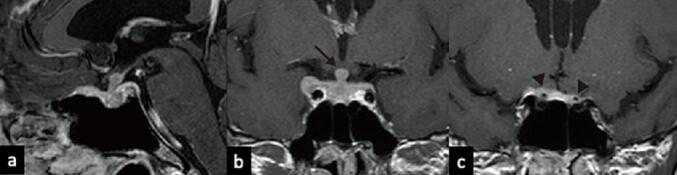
T1-weighted image with contrast before treatment demonstrates the intrasellar tumor without the optic chiasm compression (arrow) (a: sagittal, b: coronal). Coronal T1-weighted image with gadolinium contrast showing optic nerve compression (arrowhead) by the tumoral invasion of the dura (c).
